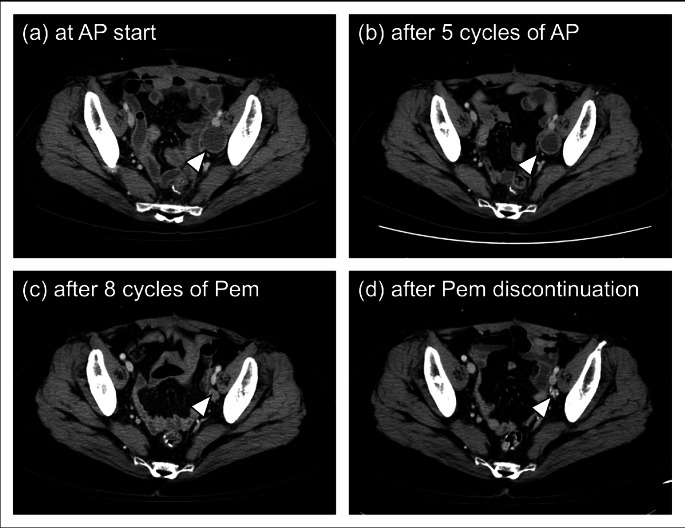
Serial CT images showing changes in the left obturator node (a) at AP start: The left obturator node was enlarged (white arrowhead). (b) after 5 cycles of AP: After five cycles of AP, the left obturator node (white arrowhead) showed minimal change in size, and the response was assessed as stable disease (SD). (c) after 8 cycles of Pem: After eight cycles of pembrolizumab, the left obturator node (white arrowhead) had markedly decreased in size. (d) after Pem discontinuation: The left obturator node (white arrowhead) remained decreased in size after discontinuation of pembrolizumab. CT, computed tomography; AP, doxorubicin/cisplatin; SD, stable disease; Pem, pembrolizumab
